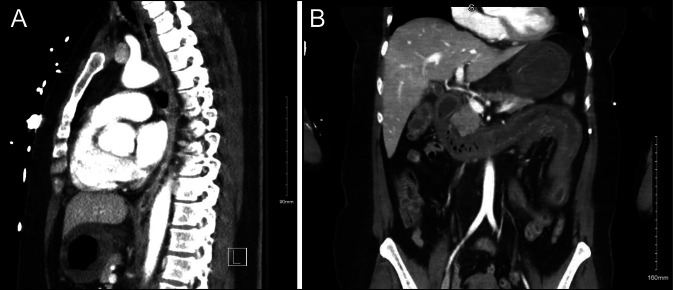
(A) CT scan showing esophageal and gastric wall thickening (sagittal plane). (B) CT scan showing gastric wall and small bowel thickening (coronal plane). CT, computed tomography.
